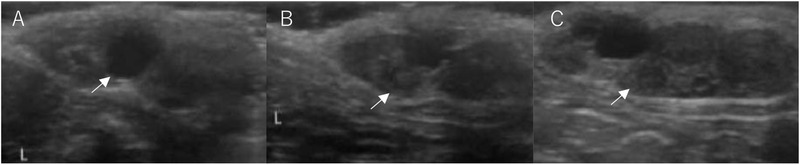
Ultrasonic appearance of the ovary clamped at the subcutaneous site on day 5 (a), 7 (b) and 13 (c) in the vaginal stimulation group. (a) The largest follicle (arrow) had a diameter of 5.8 mm. (b) The ovulated follicle (arrow) was observed after hCG treatment with vaginal stimulation by a tomcat. (c) Corpus luteum with 5.5 mm in diameter (arrow) is visible as a hypoechoic structure
